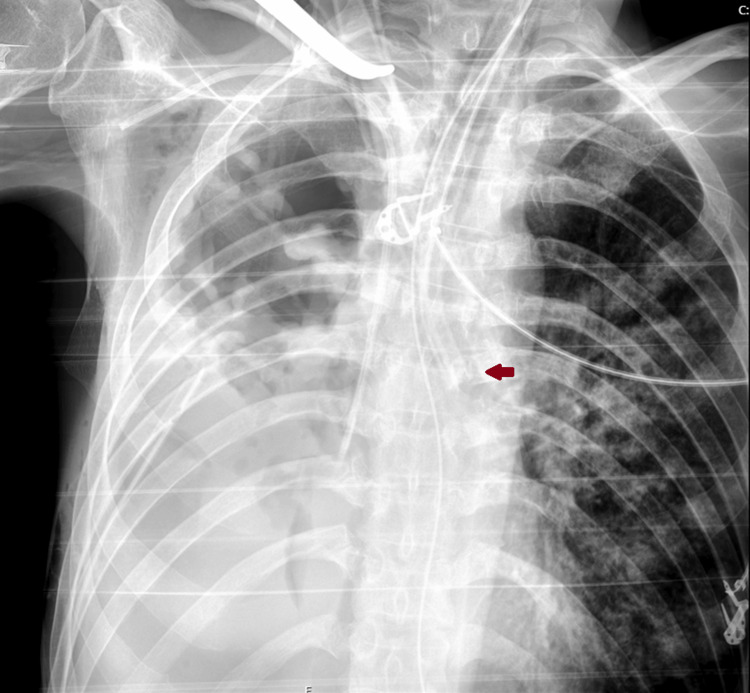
Chest radiograph during SLV Chest radiograph showing correct placement of a left-sided double-lumen tube (red arrow). SLV, single-lung ventilation
